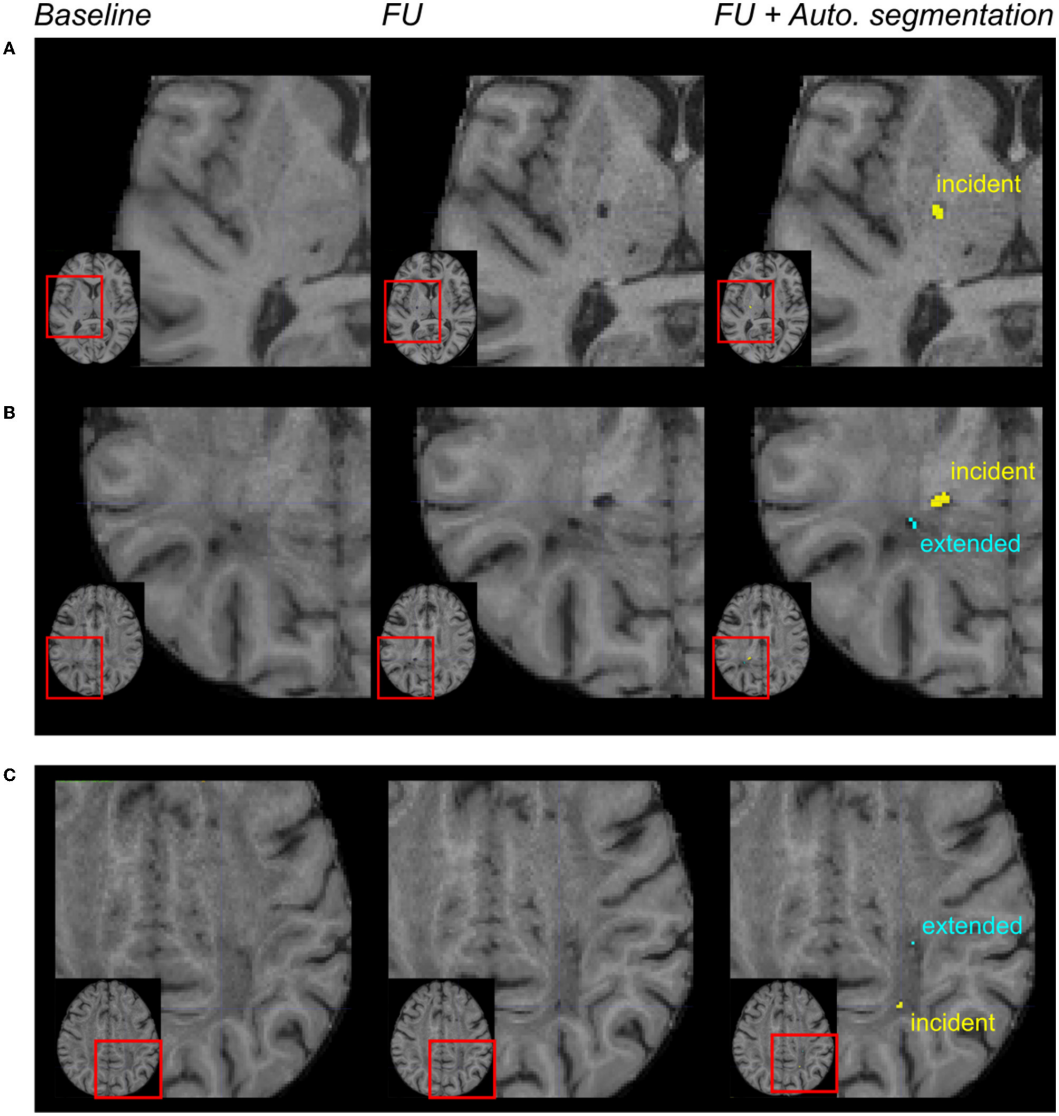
Automated incident lacunes segmentation and limitations. From left to right: magnification of MR images in different patients after the registration of the baseline and follow-up (FU) data in the MNI space. In the right column, the automated segmentation of lesions was superimposed on the FU MRI. In (A, B) incident lacunes were both identified by the experts and the algorithm. The automated method enabled to differentiate the incident lacune from the extension of a previous lacune (B). In (C) these incident lacunes were only identified by the algorithm. The automated method enabled to differentiate the incident lacune from the extended part of a baseline lacune.
